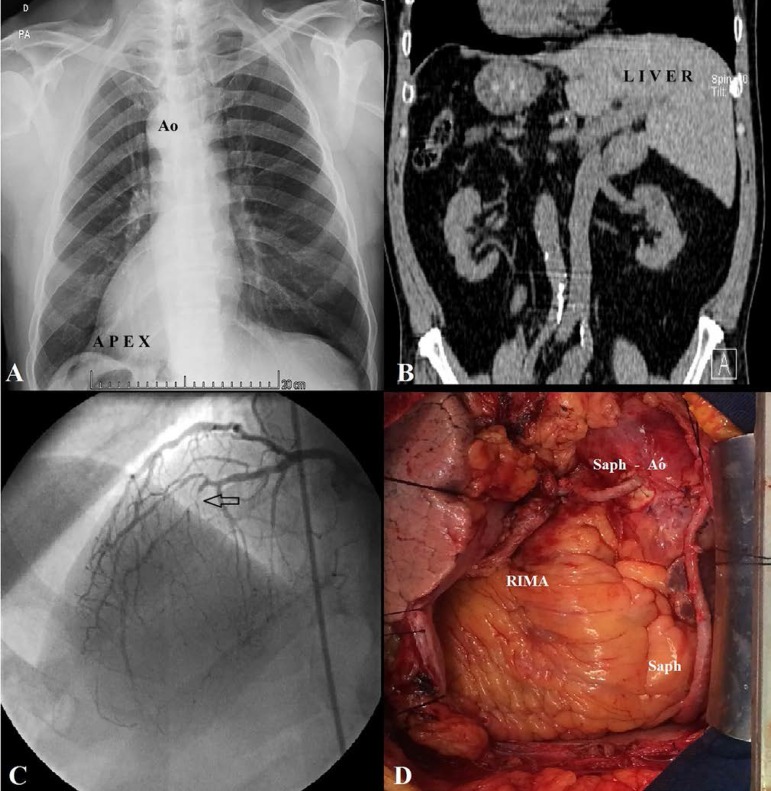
(A) Preoperative chest roentgenogram showing dextrocardia. (B) Abdominal
